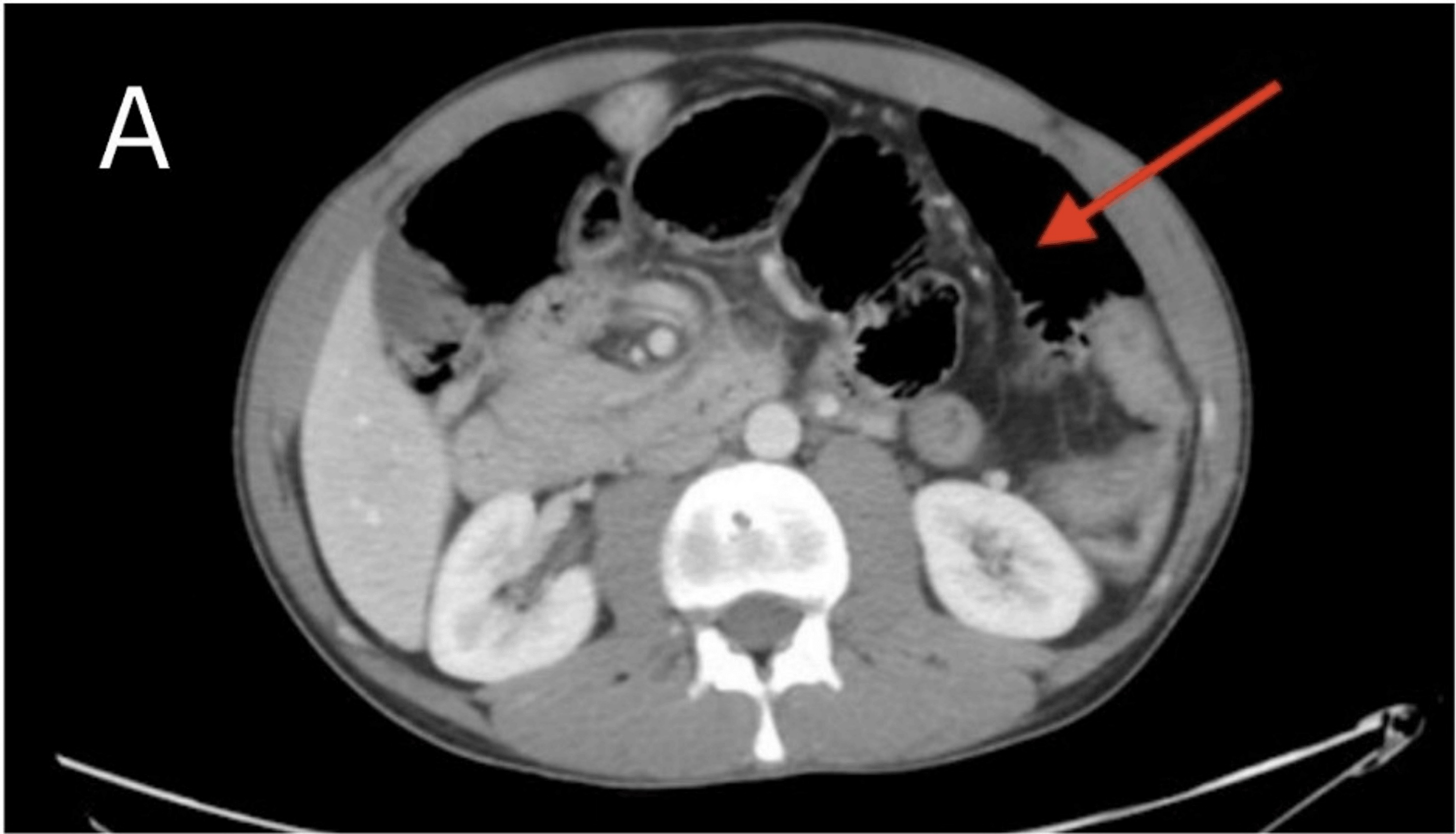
Transverse CT scan of the abdomen The transverse CT image of the abdomen further shows significant dilation of clustered small bowel loops, predominantly in the left upper quadrant, with associated air-fluid levels (red arrow). CT: computed tomography
